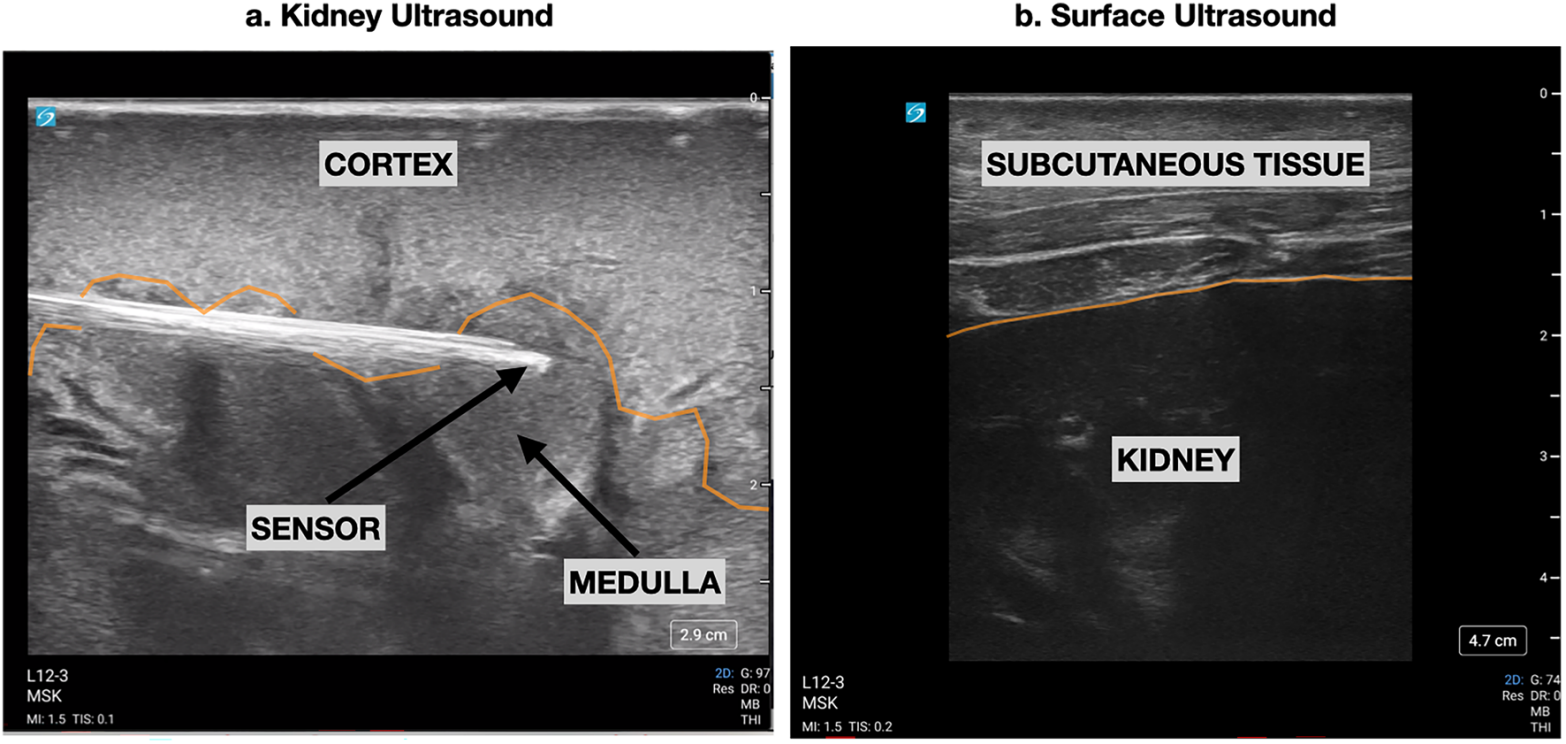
(a). Ultrasound image acquired by placing the linear transducer directly over surface of the left kidney. The 20 g IV can be seen in long axis with the tip of the oxygen sensor extending out into renal medullary tissue. (b). Ultrasound image acquired by placing the linear transducer over the skin of the left flank just below the last rib. Subcutaneous muscle and fat are seen overlying the surface of the kidney. This area was marked and a near infra-red spectroscopy sensor was placed on the skin in this location.
